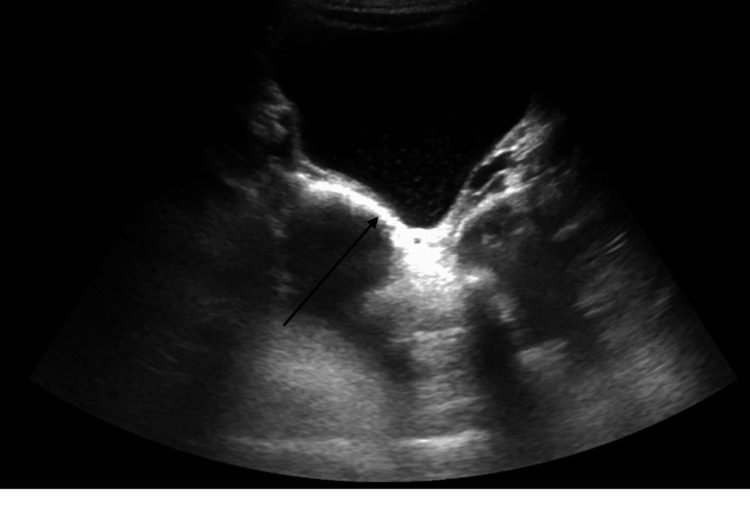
Ultrasound of the urinary bladder showing specular debris
